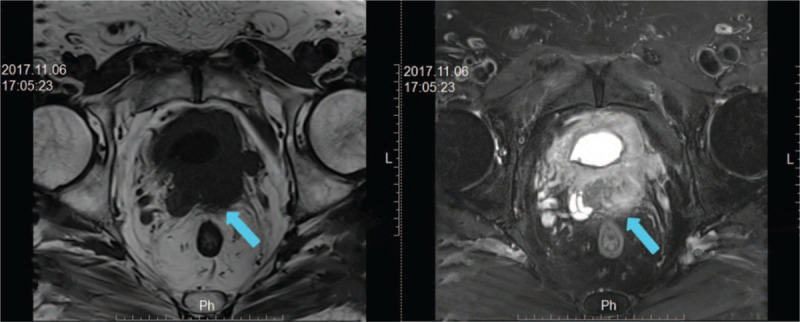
Magnetic Resonance Imaging (MRI) of the prostate revealed metastatic disease, the unclear capsule of the prostate, bilateral seminal vesicle invasion, rectal involvement, and multiple pelvic lymphadenopathies after transurethral prostatectomy and before medical treatment.
